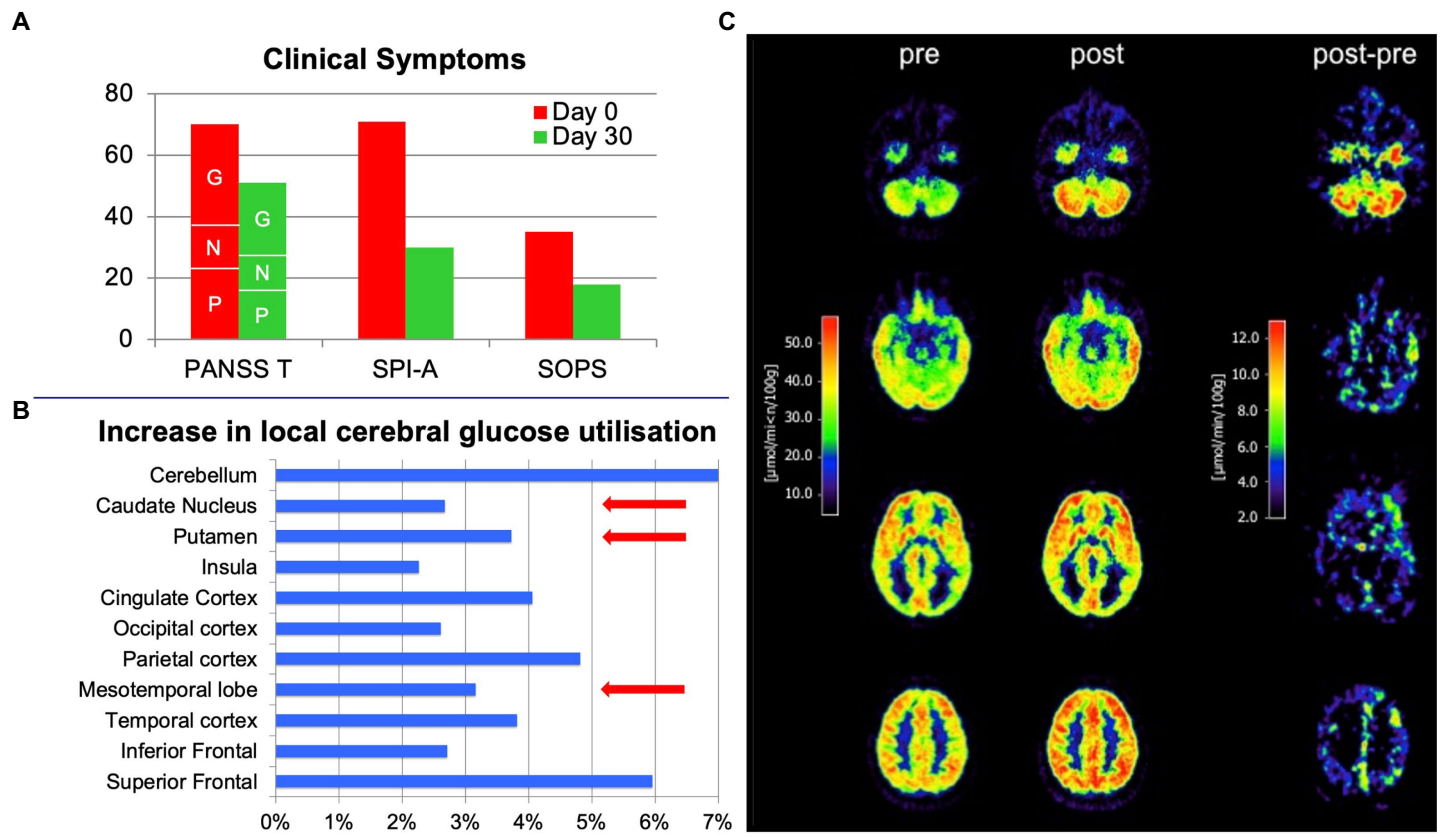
Effects of treatment with 600 mg oral cannabidiol per day in a 19-year-old male with clinically high mental risk before (pre) and on day 30 (post). (A) Positive and Negative Symptoms Scale (PANSS; P: Positive, N: Negative, G: General subscore segments marked by respective letters), the Schizophrenia Proneness Instrument - Adult (SPI-A) and the Structured Interview for Prodromal Syndromes (SIPS) before (red bars) and after (green bars) treatment. Significant clinical improvement was observed, reflected by the substantial reduction in scale scores. (B) FDG positron emission tomography scans of the brain before (pre) and after treatment (post) and the difference between post- and pre-treatment. (C) Increase in local cerebral glucose utilization after treatment in specific brain regions (in %). Brain regions (striatum, medial temporal cortex and midbrain) previously reported to be functionally modulated in CHR individuals by a single oral dose of 600 mg cannabidiol in a functional MRI study (11) are highlighted by red arrows.
